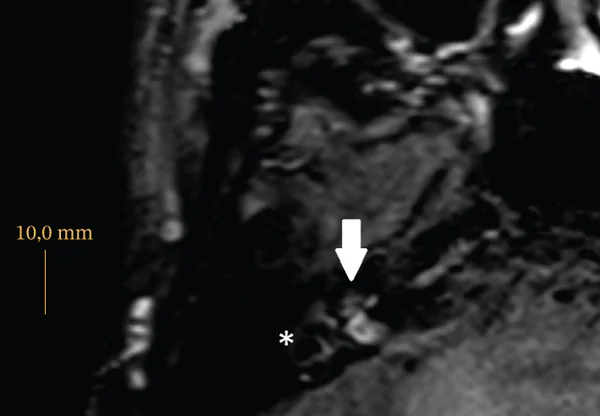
Axial section of the 3D FLAIR sequence of an MRI at the first time point without ELH in the vestibulum (white star) or in the cochlea (white arrow) with a scale bar.
